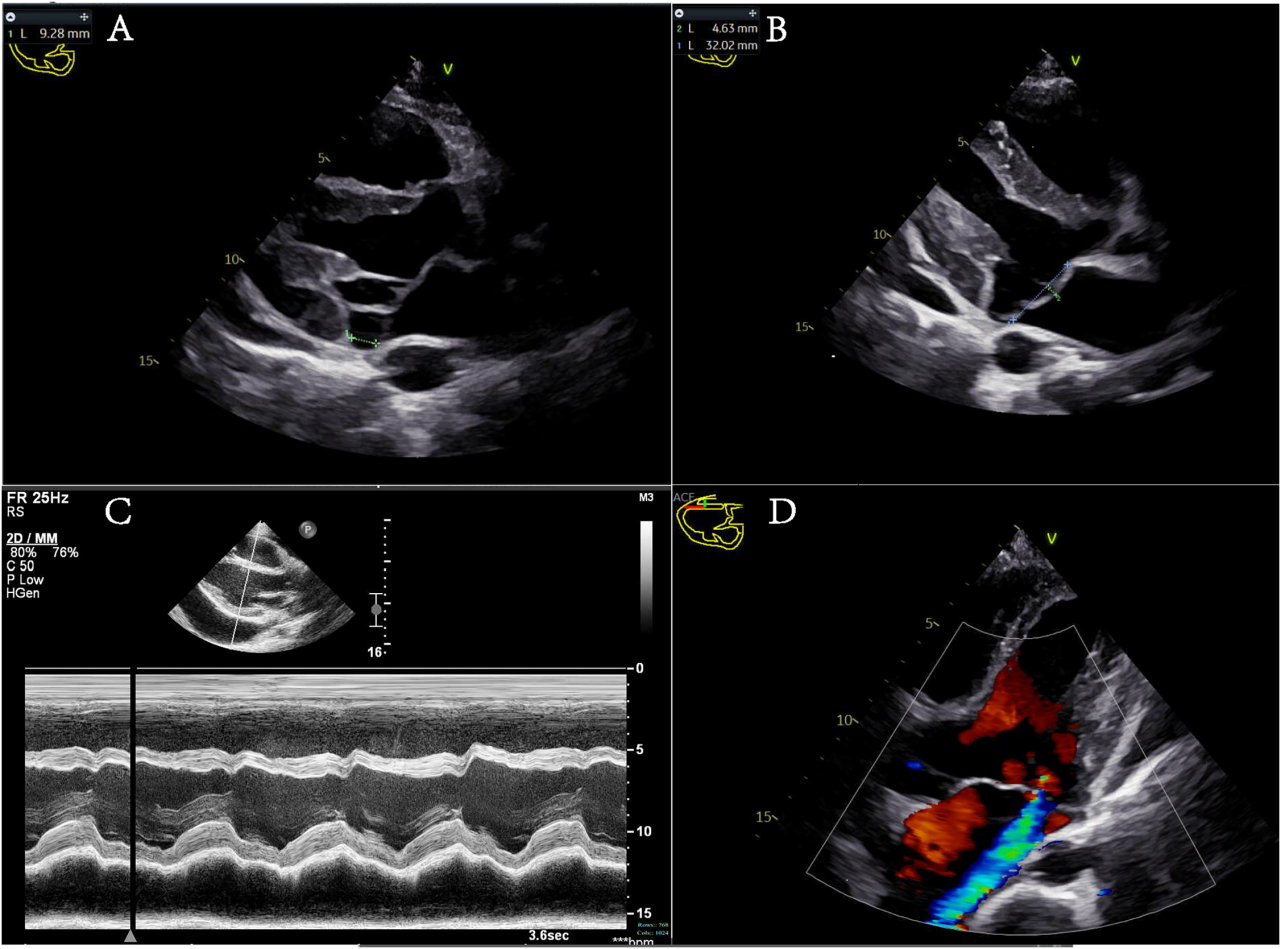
(A–C) Different views of the parasternal long-axis section of the left ventricle. (A) Mitral annular disjunction during systole, with a green dashed line indicating the separation distance of 9.28 mm. (B) The anterior leaflet of the mitral valve protrudes into the left atrium during systole, with a protrusion distance of 4.63 mm. (C) An M-mode echocardiogram displaying the ventricular wave complex. Left ventricular systolic function is normal, with signs of a pericardial effusion. (D) The apical five-chamber view showing mitral valve prolapse with moderate regurgitation.
